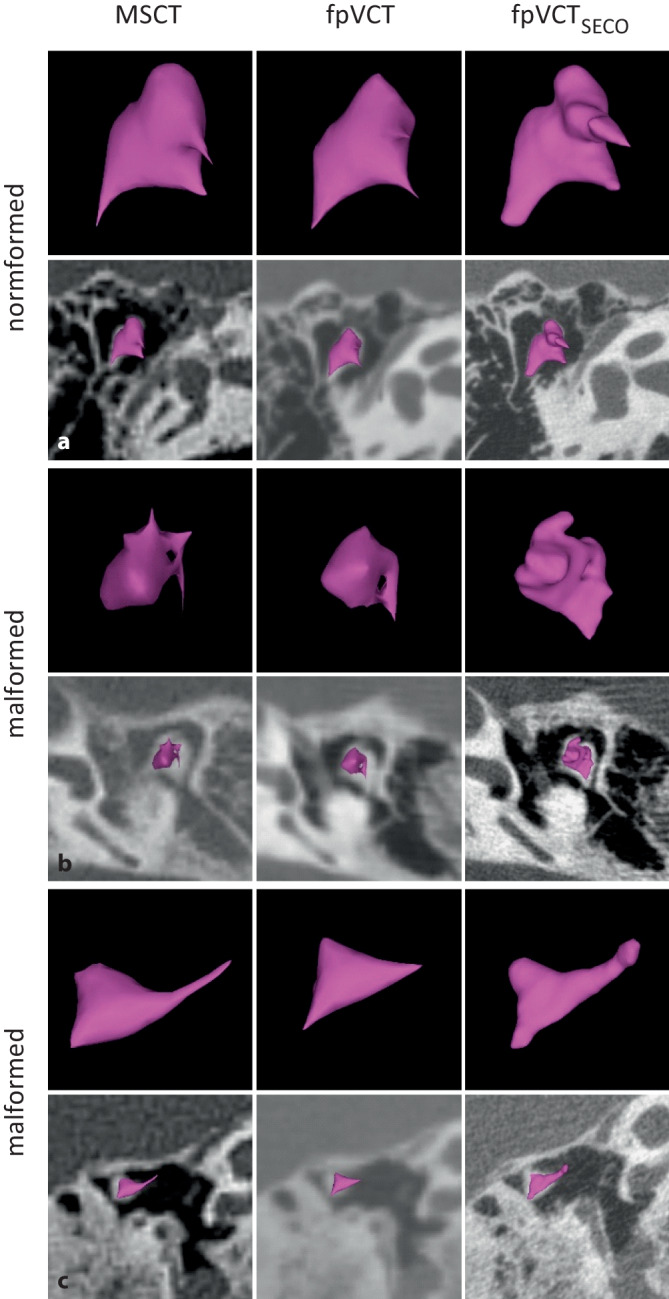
3D visualization of the malleus–incus complex in the OTOPLAN planning software® using MSCT (600 µm slice thickness), fpVCT (466 µm slice thickness), and fpVCT_SECO_ (99 µm slice thickness). The complexes are each shown in isolation and with an overlaid axial plane. A normally configured complex a, a moderately malformed complex b, and a severely malformed complex c are shown. *MSCT* multislice computed tomography, *fpVCT* flat-panel volume computed tomography, *fpVCT*_*SECO*_ secondary reconstructions of fpVCT
